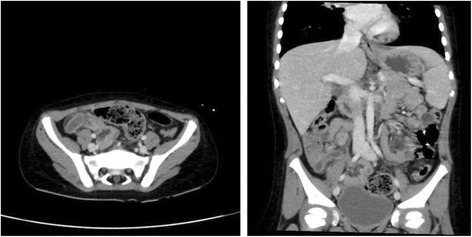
Cross section and coronal view CT scan for the same patient shows prove the diagnosis of acute appendicitis
